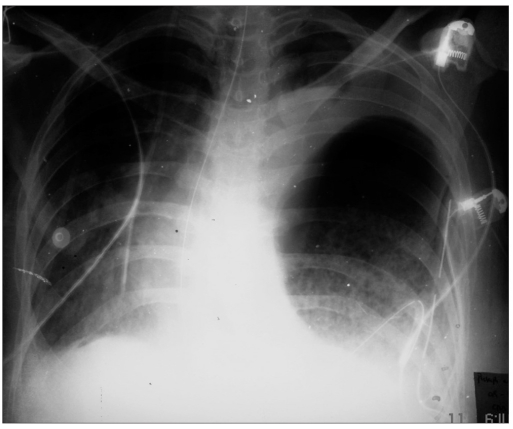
Chest X-ray showing a distended stomach in left hemithorax and contralateral mediastinal shift. Left lung is compressed
